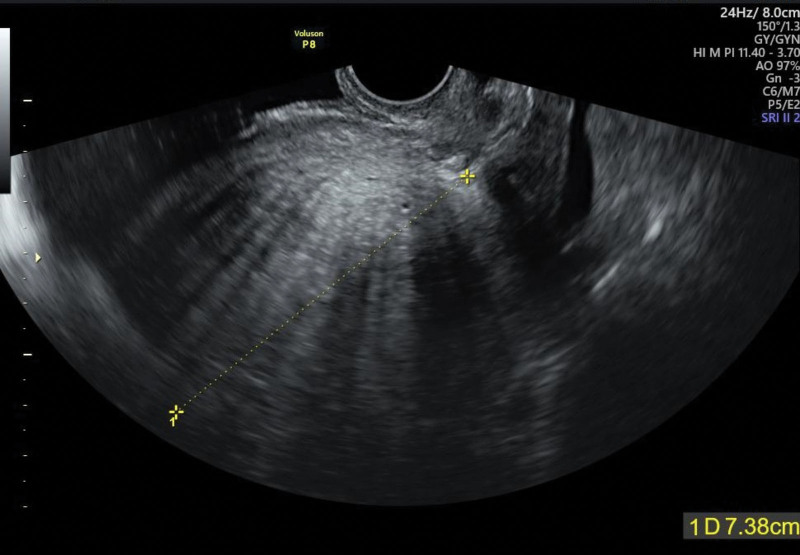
Uterine ultrasound. Note the thickening of the uterine wall and the relatively uniform echogenicity.
